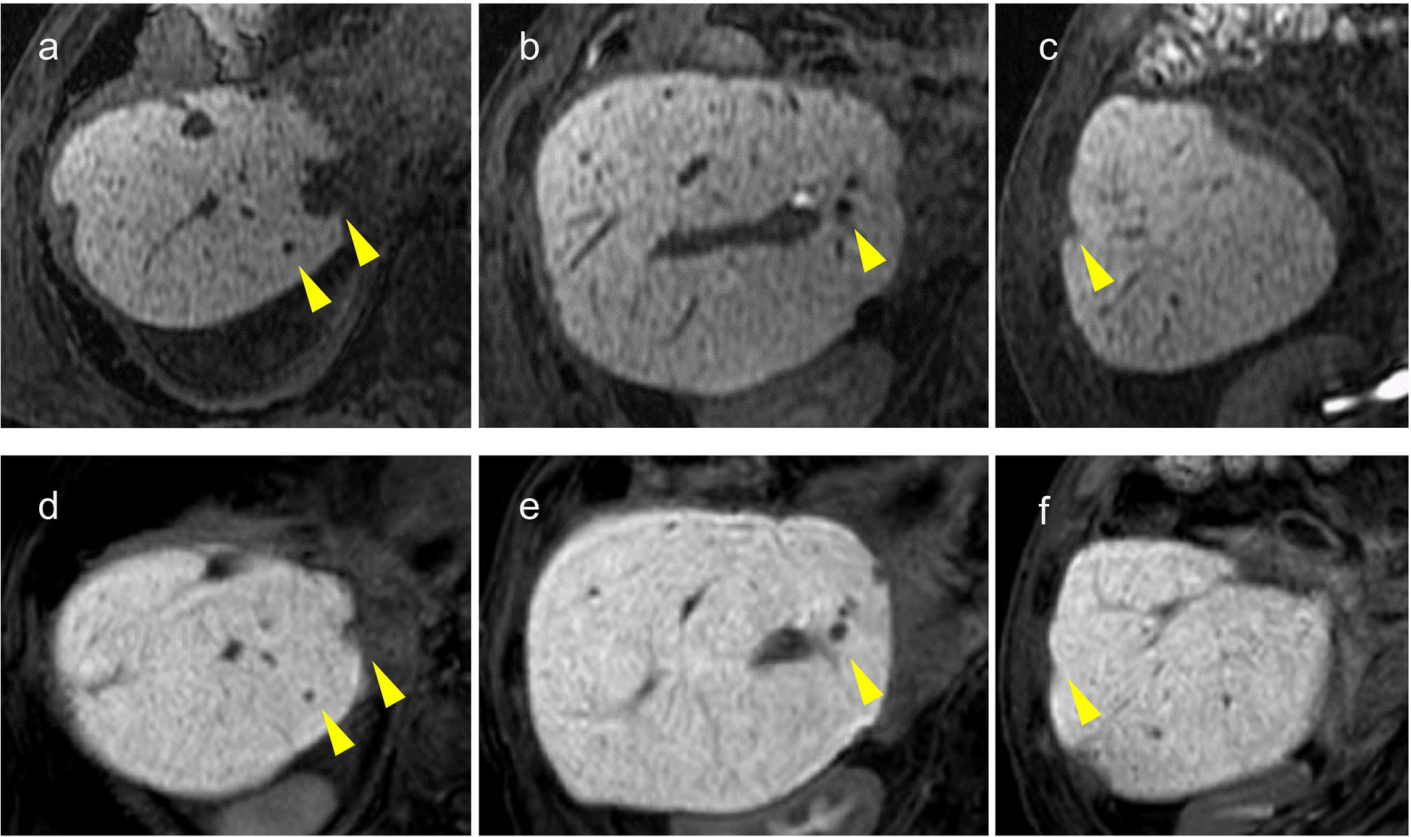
Third recurrence. a–c Four liver metastases are detected by EOB-MRI. d–f All tumors shrunk after 6 months of everolimus (10 mg/day) administration. The arrows point the liver metastases detected by EOB-MRI
